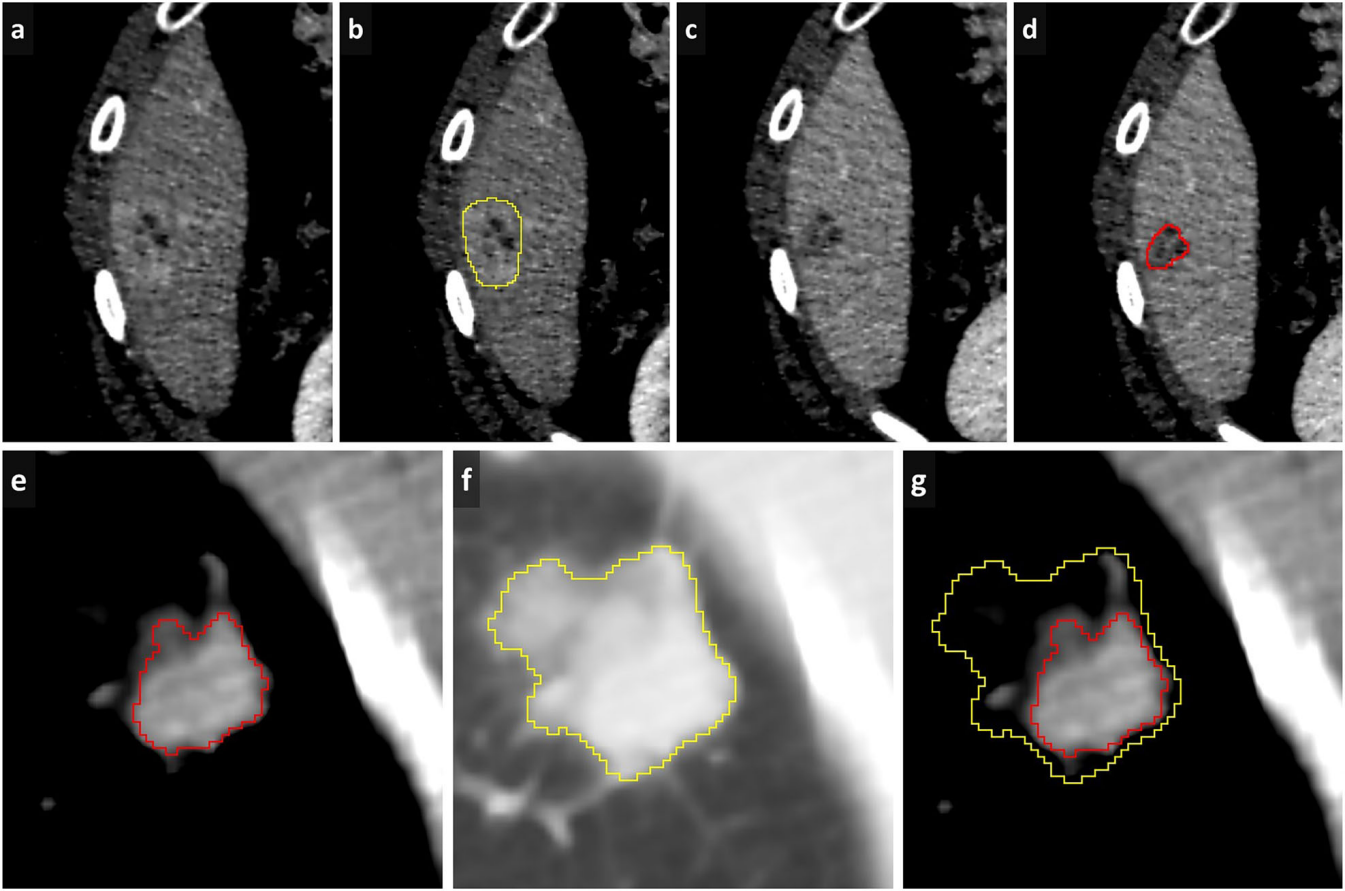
a–d Axial CT images of a liver lesion (neuroendocrine tumor metastasis) in the arterial (a, b) and portovenous (c, d) phases, showing differences in segmentation size and shape, with calculated volumes of 6.7 cm³ and 3.0 cm³, representing a 55% variation. e–g Segmentations of a lung lesion using soft tissue (e) and lung (f) windows, with a comparison of both segmentations shown in (g). Volume calculations reveal a significant difference—4.7 cm³ vs 10.8 cm³—resulting in a 56% variation
